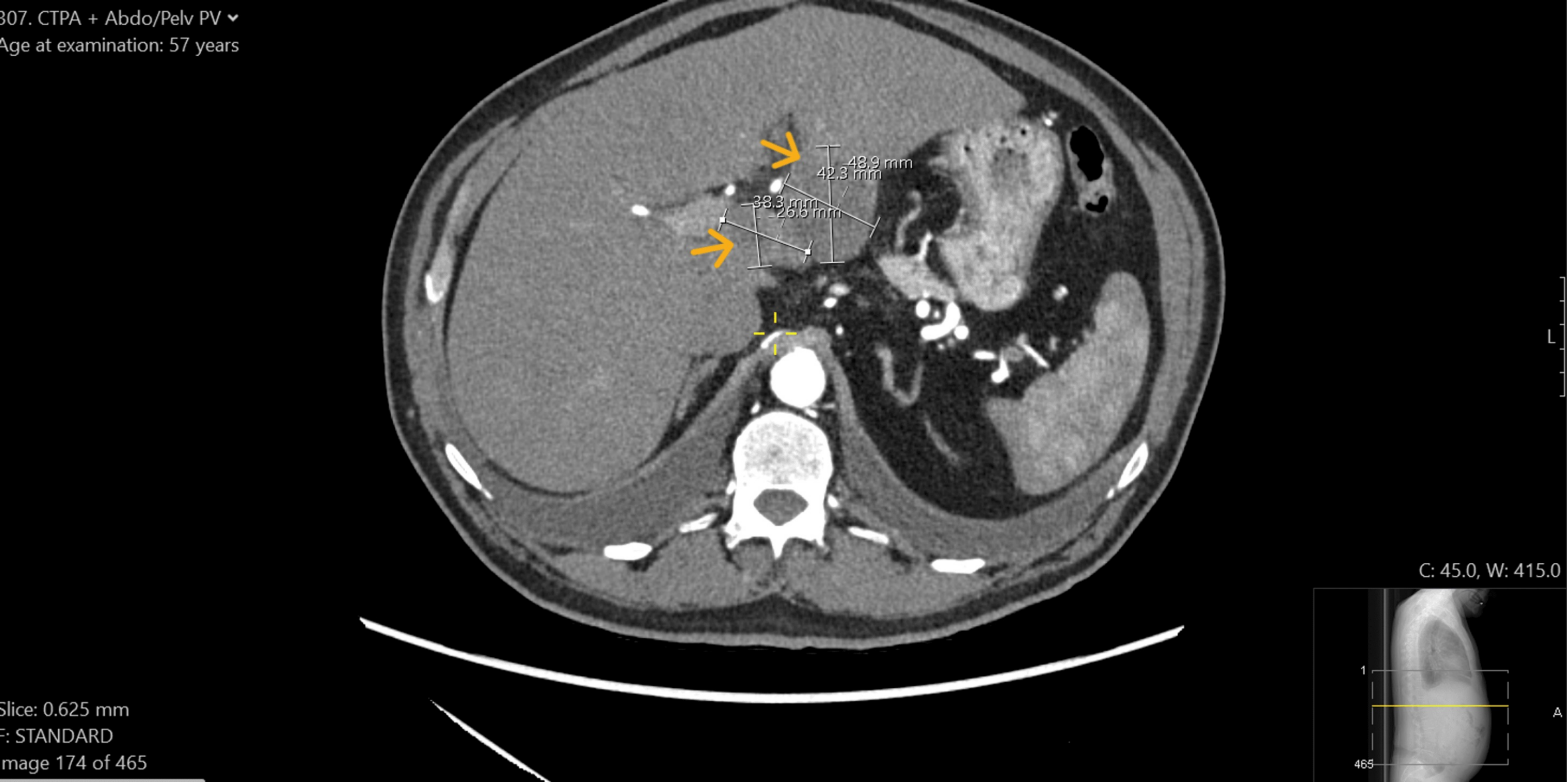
CT abdomen and pelvis Arrow indicating porta hepatis lymphadenopathy (left), and arrow indicating a mass in the left liver lobe (right).
